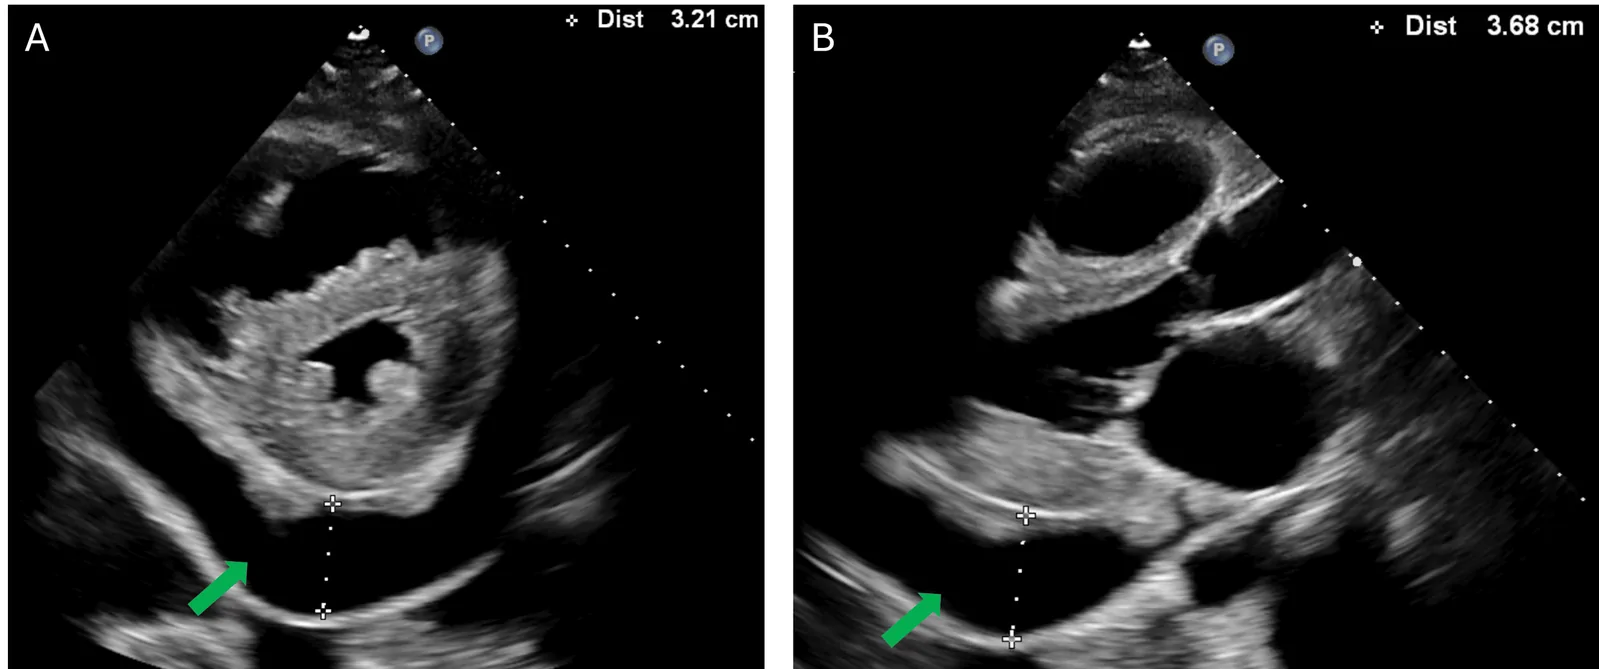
Multiple echocardiographic views of the large pericardial effusion. (A) Parasternal short-axis and (B) parasternal long-axis views of the large pericardial effusion measuring >3 cm (as denoted by the green arrows). The posterior location of the effusion meant there was no window for percutaneous drainage.
